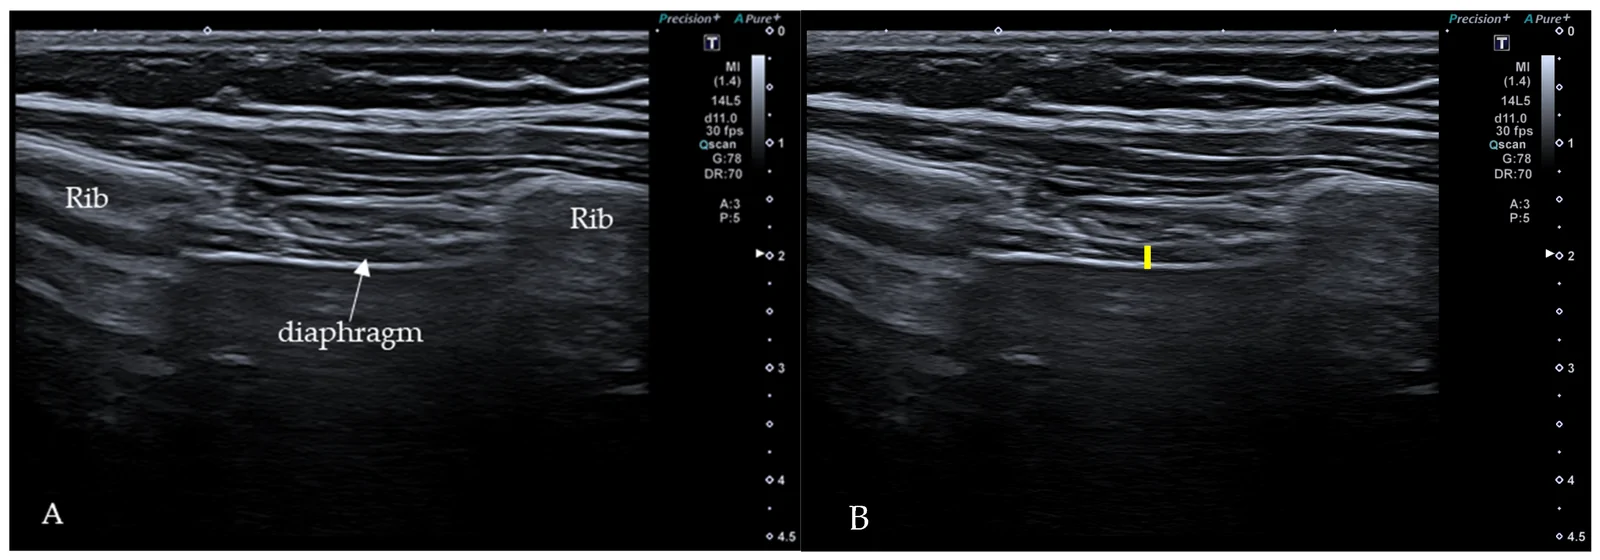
(A) Representative B-mode ultrasound image of the diaphragm obtained with a linear transducer over the lower intercostal space on the midaxillary line. (B) Measurement of diaphragm thickness at the zone of apposition. The diaphragm appears as a hypoechoic layer between the pleural and peritoneal hyperechoic lines. Yellow calipers indicate the distance used to quantify diaphragm thickness.
